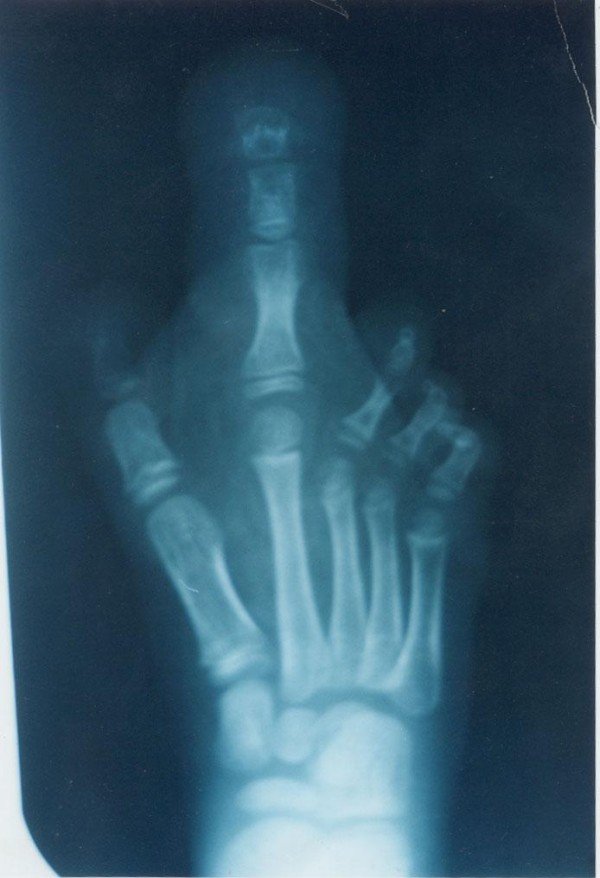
X-ray image showing hypertrophy of the soft tissues of the 2^nd ^digit of right foot. The phalanges of the involved digit also appear enlarged.
